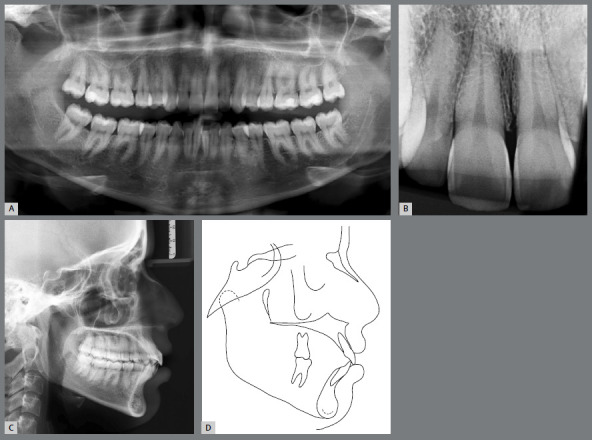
Initial panoramic radiograph (A), periapical radiograph (B), lateral cephalometric radiograph (C) and cephalometric tracing (D).
